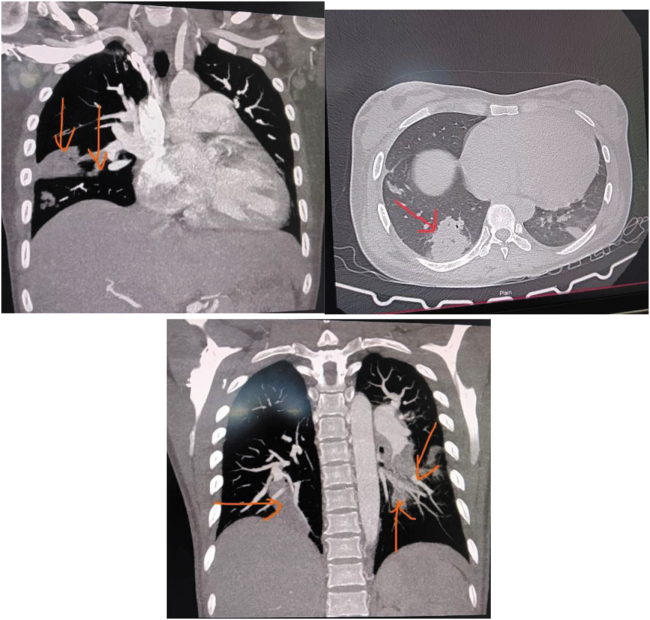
Chest X-ray showing opacification in the mid and lower zones of the right lung, PA view.
